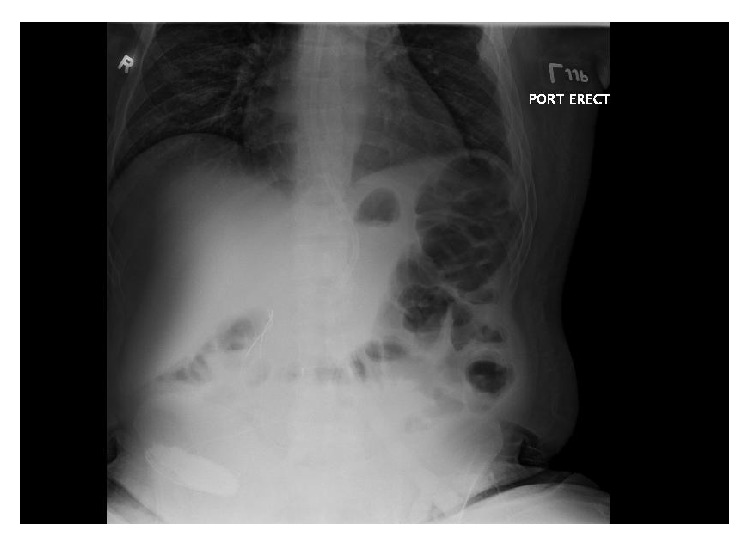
Portable chest radiograph demonstrating jejunostomy tube extending retrograde through esophagus, with no evidence of perforation.
